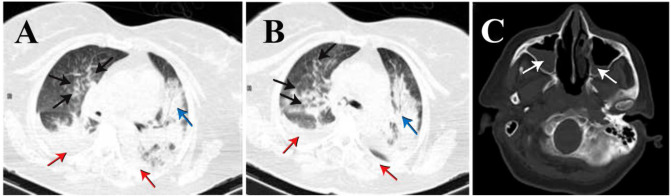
Computed tomography of the lung; Consolidation (Acinar shadow) (blue arrow) with peripheral ground-glass opacities in the lower lobe of both lung (black arrows) and bilateral mild pleural effusion (red arrows) (A and B); Computed tomography of the brain; bilateral sinuses opacification with air-fluid levels (white arrows) (C)
